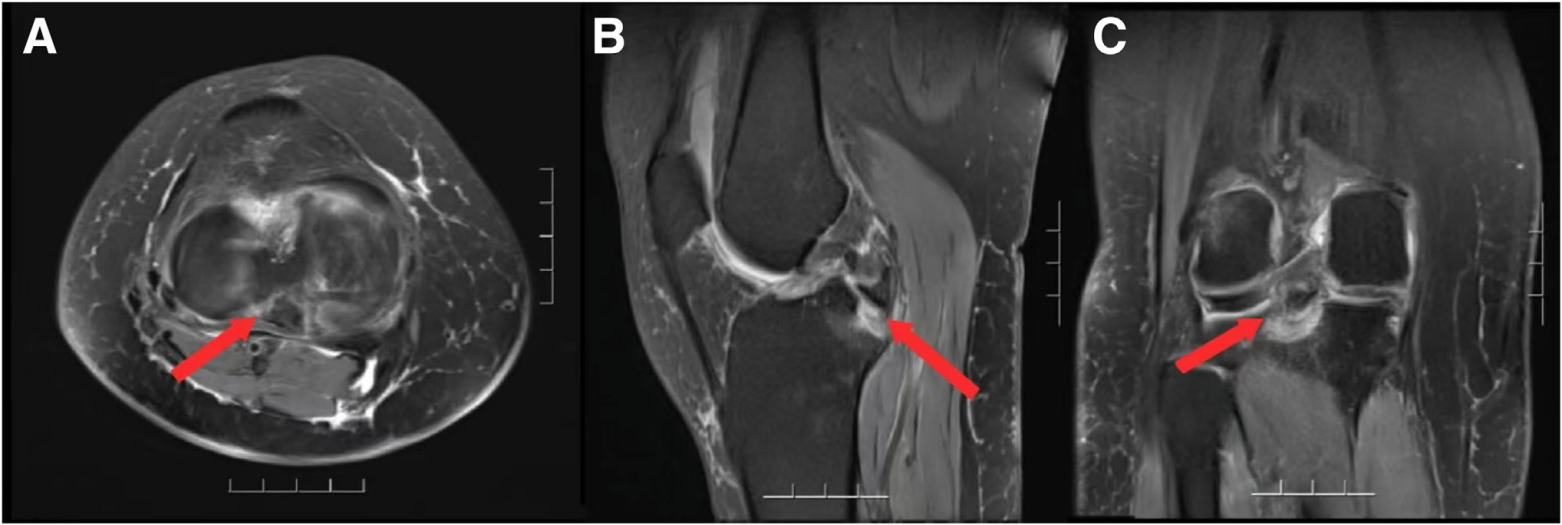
Magnetic resonance imaging scans of the patient before surgery (patient side: right). (A) Transverse plane. (B) Sagittal plane. (C) Coronal plane.
